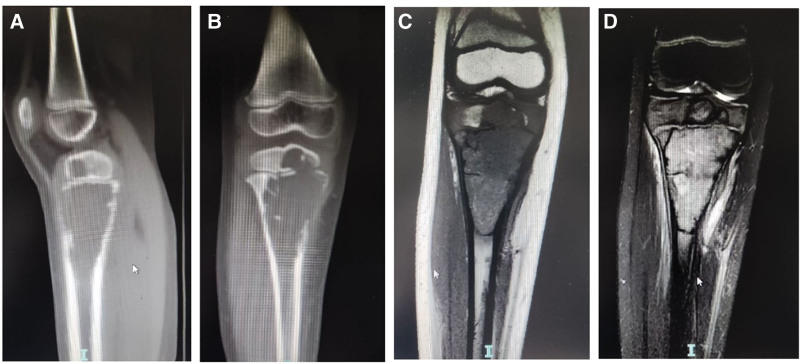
CT sagittal plane (A) and coronal plane (B) showed distended osteolytic bone destruction of the right proximal tibia metaphysis and bone, with mild sclerosis of part of the margins, bone crests on the edges, localized interruption of the bone cortex, and swelling of the surrounding soft tissues. t1 image (C) and t2 image of MRI showed bone destruction foci of the right proximal tibia, which was slightly distended with a clear boundary, sclerosis of part of the margins, involvement of the metaphyseal end and epiphysis, and localized disruption of the adjacent bone cortex. The lesion is about 6.8*3.5*3.1 cm (upper and lower + left and right + anterior and posterior), with T1WI equal and T2WI high signal, accompanied by multiple bone crests. Diffuse edema of soft tissues around the lesion. The right fibula did not show clear signs of bone destruction.
